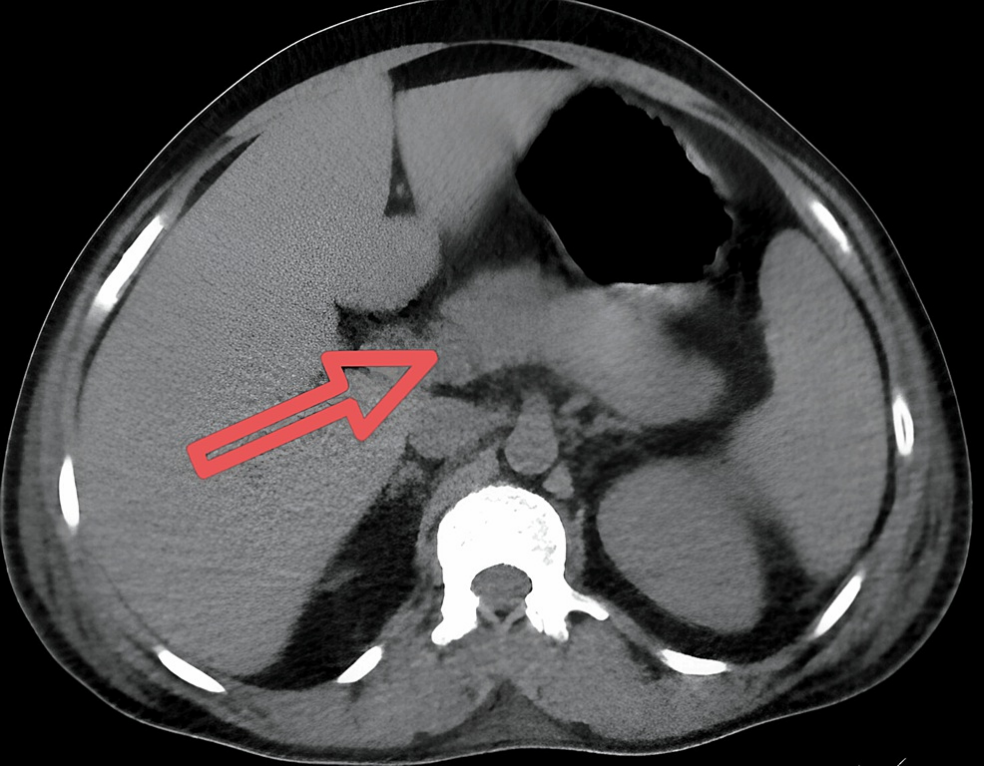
CT of the abdomen showing bulky pancreas
